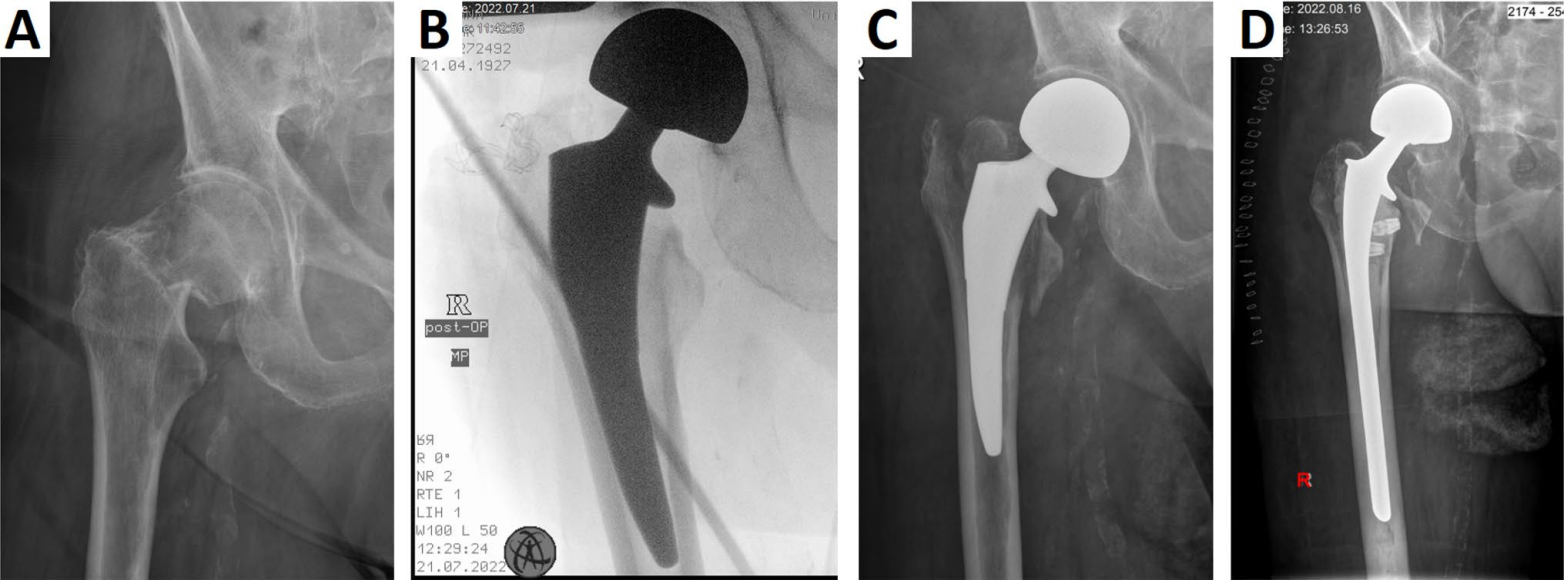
(a) X-rays showing a case of femoral neck fracture in a 95-year-old man. An additional medial cortical bone defect can be observed in intraoperative luminescence, (b) probably caused by excessive osteotomy. (A rather secondary, but definite reason for a weak calcar) (c) X-ray due to occurring pain during mobilization 10 days after surgery revealed a fracture of the major and minor trochanter region, leading to instable implant. (d) Revision was performed using a cemented long stem, allowing immediate mobilization of this frail patient
